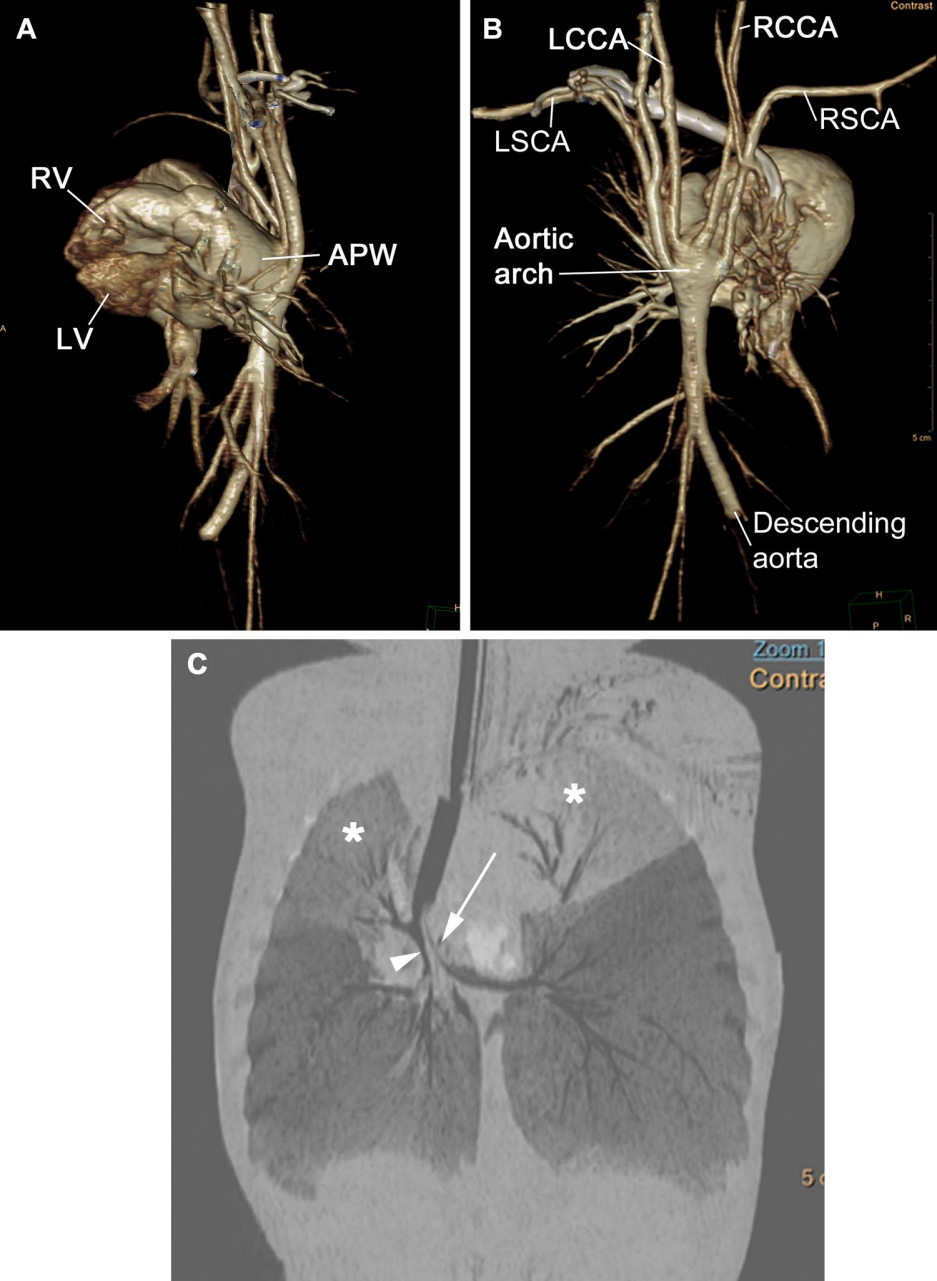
MDCTA reconstructed images. A Lateral 3DVR demonstrating superior RV and inferior LV with an APW. B Posterior 3DVR reveals four elongated head and neck arterial branches from the posteroinferior aortic arch and abrupt high termination of the abdominal descending aorta. C Coronal MinIP (lung window) reconstruction showing ribbon-like stretched right bronchus intermedius (arrowhead), collapsed proximal left main bronchus with bilateral upper lobe atelectasis (*), and ball-valve type hyperinflation of the remainder of the lungs. *MDCTA* multidetector CT angiogram, *3DVR* three-dimensional volume rendering, *APW* aortopulmonary window, *LCCA* left common carotid artery, *RCCA* right common carotid artery, *LSCA* left subclavian artery, *RSCA* right subclavian artery, *MinIP* minimum intensity projection, *superior RV and inferior LV* superinfero ventricles
